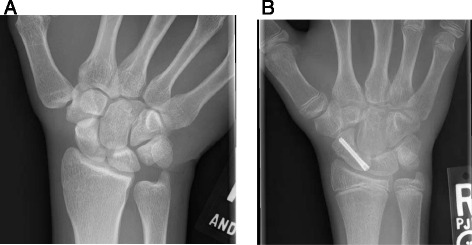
a PA radiograph of a nondisplaced proximal pole scaphoid fracture in a recreational hockey player. b PA radiograph of a nondisplaced scaphoid waist fracture in a high school soccer player treated with headless compression screw fixation
